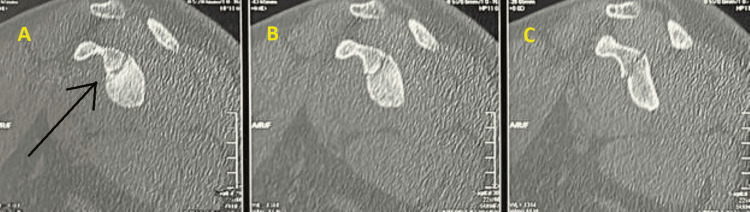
Repeat CT scan showing callus formation A)-C) Serial axial CT cuts of the left shoulder joint The black arrow shows callus formation
